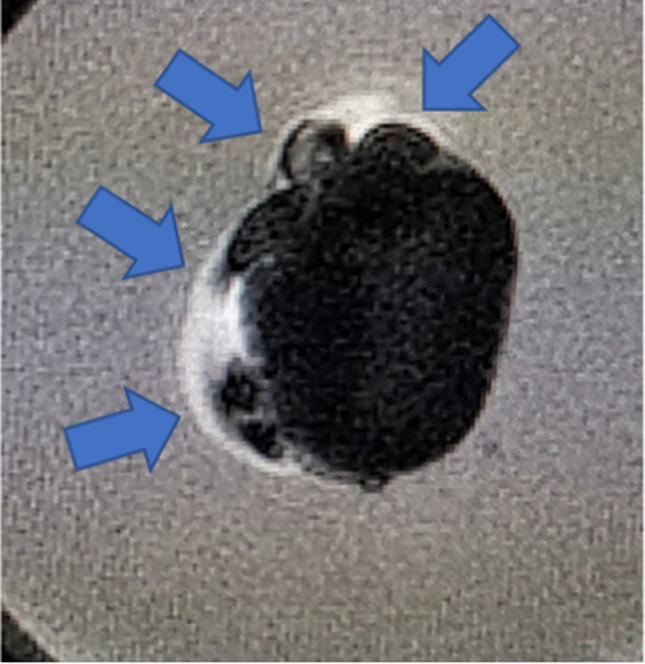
Tooth 31, transversal view, MRI dataset. Susceptibility of artifacts at the junction of the tooth and embedding material (blue arrows)
